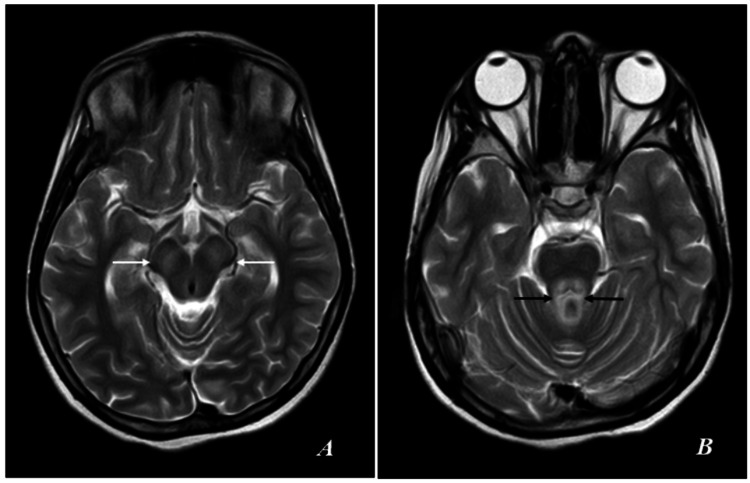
MRI of the brain. (A, B) T2-weighted axial image showing a combination of the “face of a giant panda sign” (short white arrows) and the “face of a miniature panda sign” (short black arrows) refers to the “double panda sign.”
